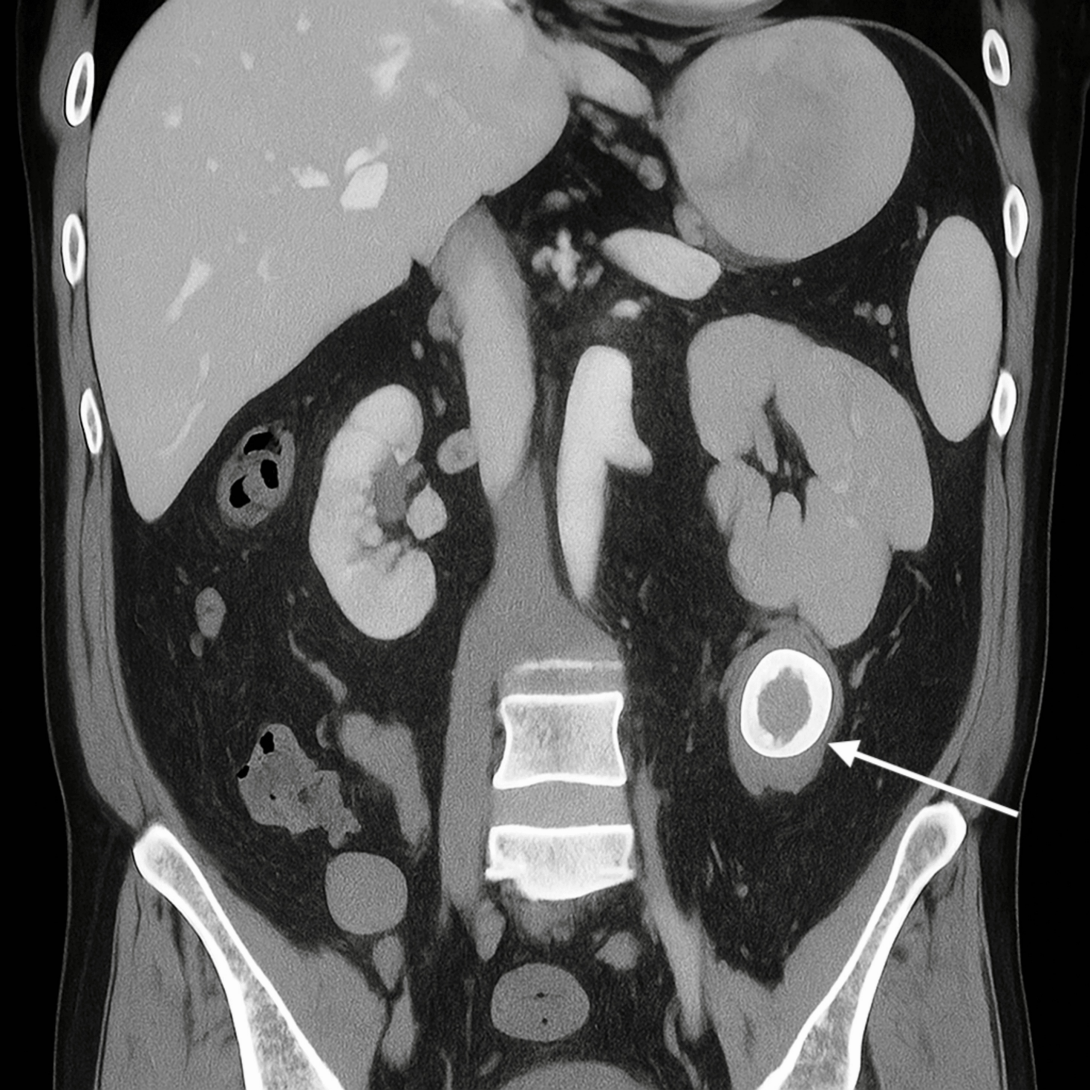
CT coronal view showing ectopic gallstone (arrow). Coronal CT image demonstrating the same ectopic gallstone (white arrow) with surrounding bowel dilatation. A cholecystoduodenal fistula is suspected based on adjacent wall thickening. Scale bar = 1 cm.
